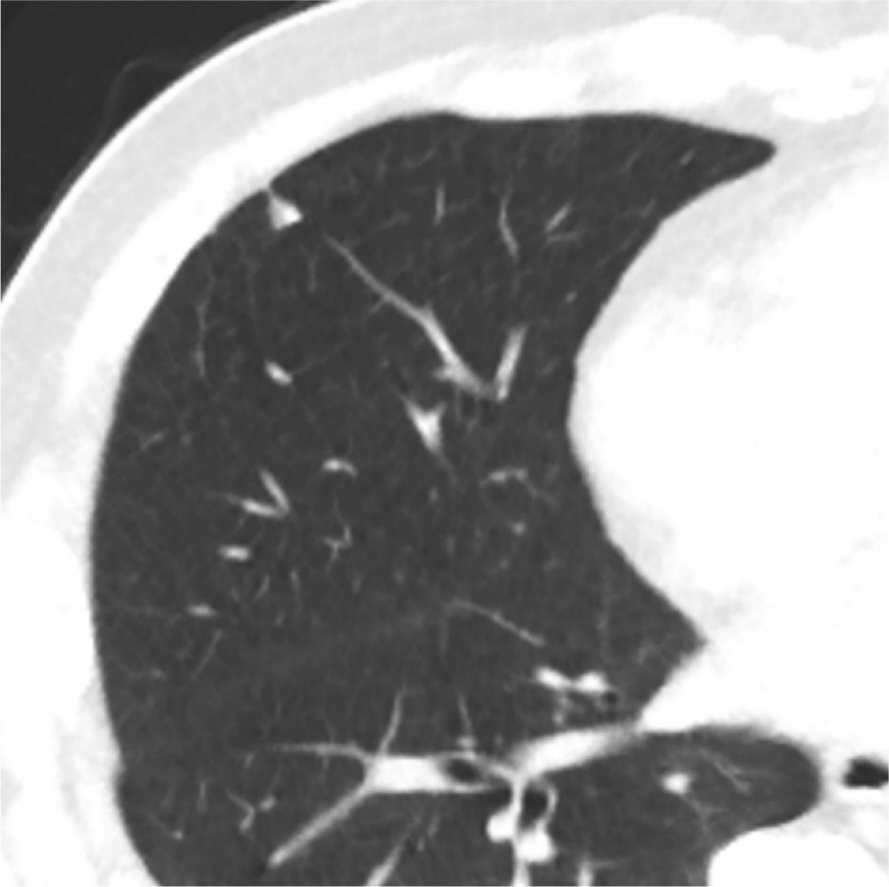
Enlargement of the intrapulmonary lymphatic apparatus (intrapulmonary lymph nodes). Low-dose CT screening image. Shooting conditions: CTDIvol = 0.61 mGy; slice thickness: 2.5 mm; sequential approximation reconstruction (model-based iterative reconstruction: MBIR) (WW WL) = (1,200–, 600). The low-dose CT screening image shows a 6 mm triangular-shaped nodule slightly away from the pleura in the right middle lobe. Linear opacity showing interlobular septa is visible between the nodule and the pleura. The shape strongly suggests an enlargement of the intrapulmonary lymphatic apparatus
